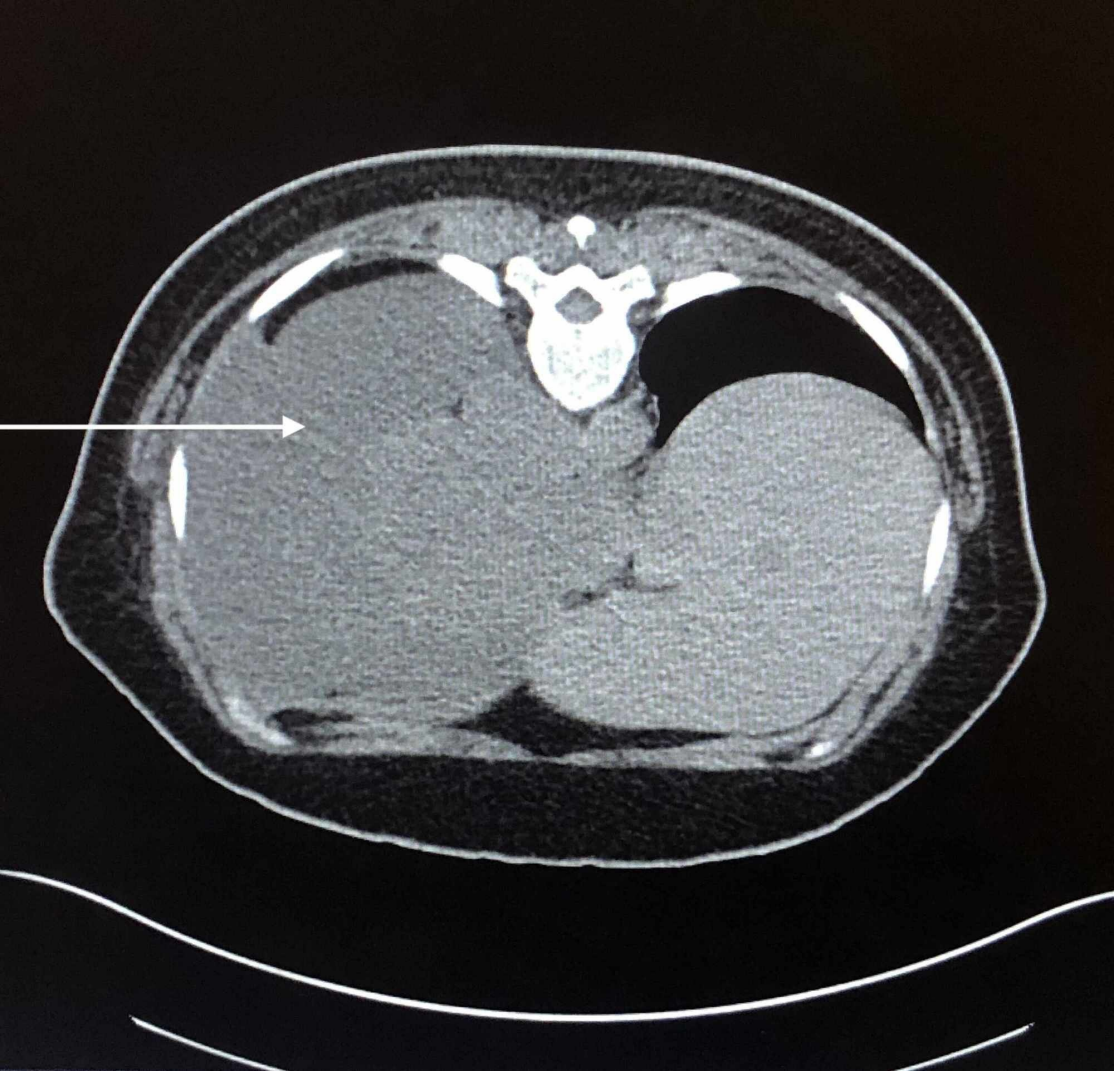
CT-guided thoracentesis and biopsy imaging shows left lung mass.
